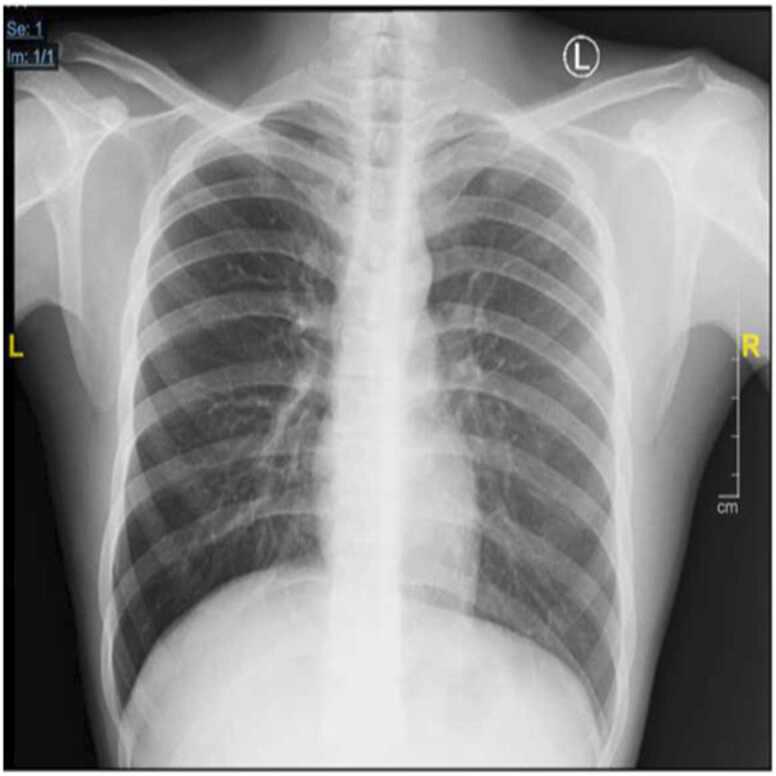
Chest X-ray on 4th month of treatment. Infiltrates below the left lung decreased.
